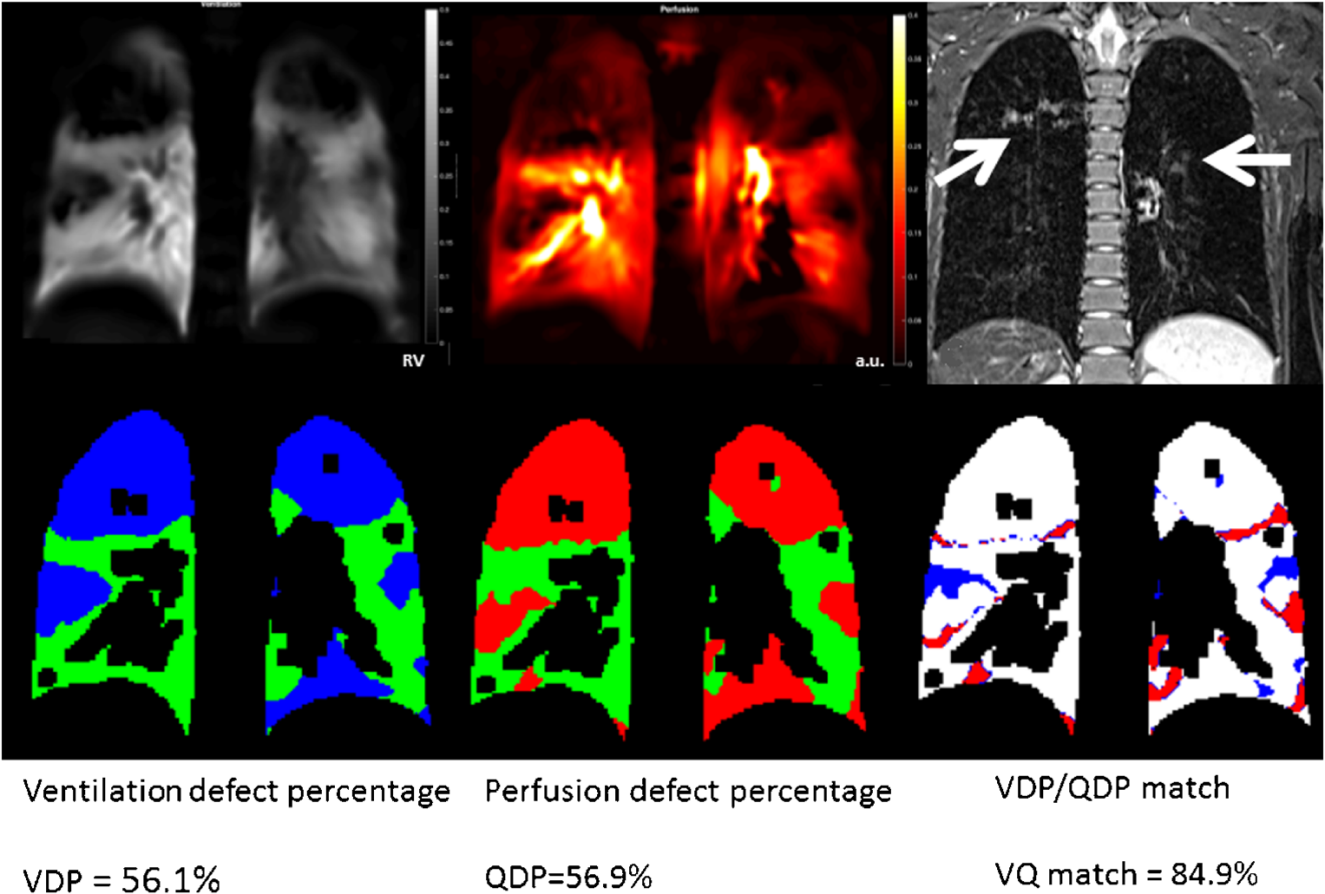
Phase-resolved functional lung (PREFUL) MRI in a 13-year-old girl with cystic fibrosis. Coronal PREFUL MRI-derived regional ventilation (*left column*) and perfusion-weighted image (*middle column*) with ventilation defect percentage (VDP, *blue*), perfusion defect percentage (QDP, *red*) and ventilation/perfusion (VDP/QDP) maps (*white,* signifying matched ventilation-perfusion). The girl has typical heterogeneous areas of hypoperfusion (*red*) and hypoventilation (*blue*), which are predominantly matched on the VDP/QDP map (*white*), mainly because of mucus plugging and bronchial wall thickening in the central upper airways (*arrows*), as shown on the corresponding turbo inversion recovery magnitude image. Movies in the Online Supplementary Material show PREFUL MRI-derived regional ventilation over the whole ventilation cycle (Movie 3) and perfusion-weighted images during the whole cardiac cycle (Movie 4)
